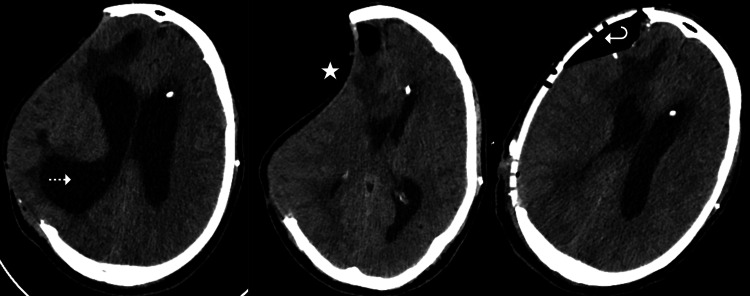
Axial view brain CT scan Dashed arrow shows hydrocephalus; star shows paradoxical brain herniation; curved arrow shows cranioplasty with no complications associated
